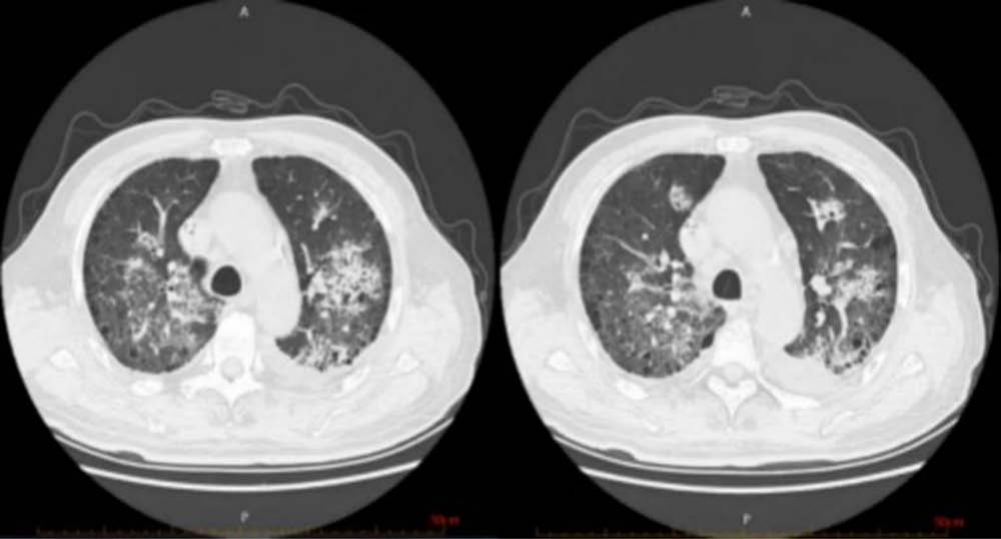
Lung CT on March 26th: compared with March 11th, multiple fibrotic streaks and exudative lesions in both lungs have significantly worsened compared before, while the patchy high-density shadow in the lower lobe of the left lung has slightly decreased compared before. CT = computed tomography.
